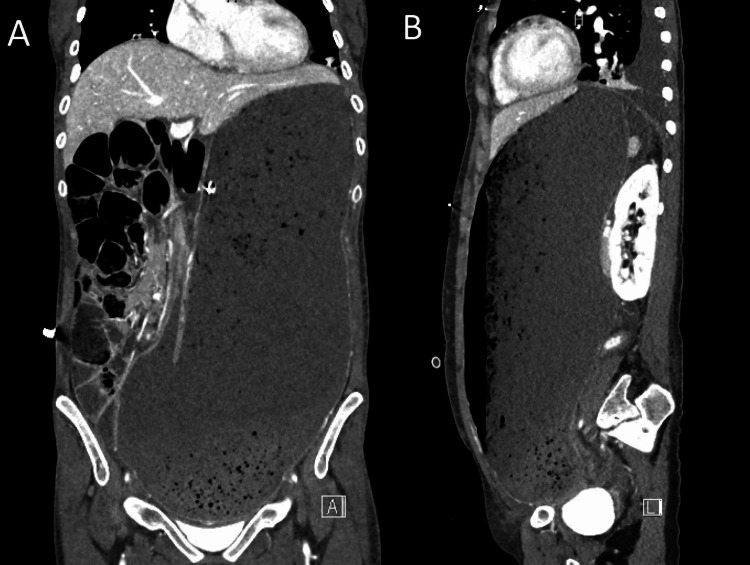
Coronal (A) and sagittal (B) abdominal CT scans showing acute gastric dilatation. The stomach is severely distended with an air-fluid level reaching the pelvic floor.
